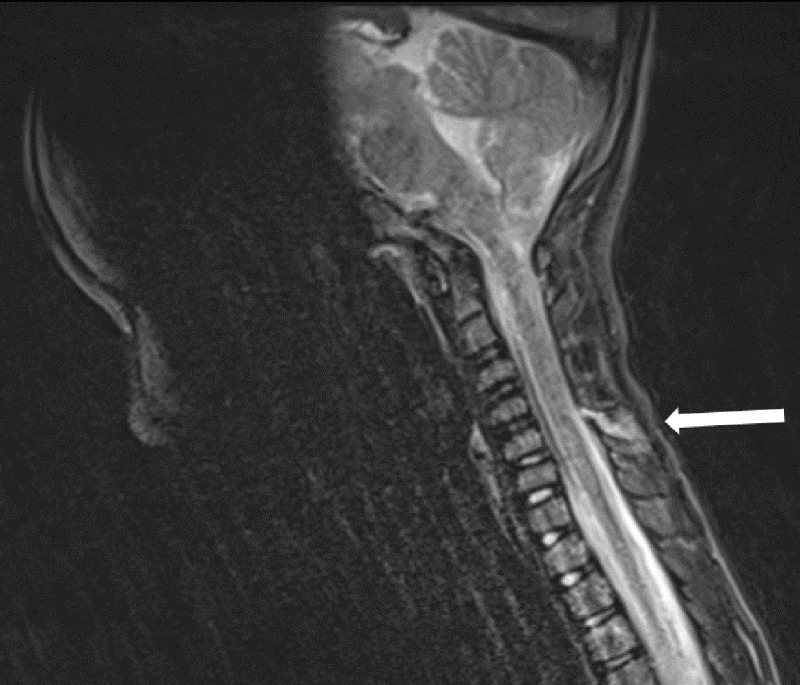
MRI showing C5-C6 interspinous ligament oedema.
